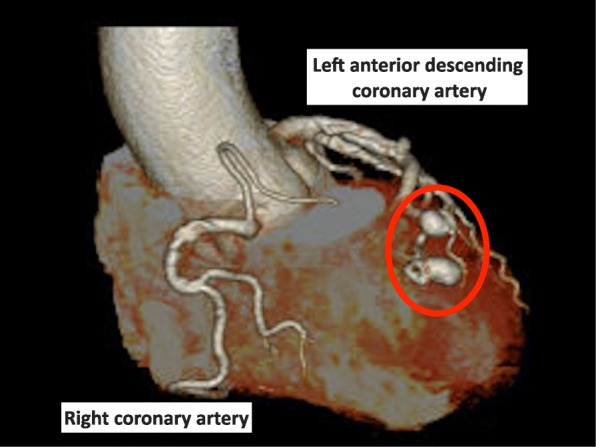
Preoperative coronary 3D-CT: Two coronary artery aneurysms are recognized in the periphery of the left anterior descending branch (red circle)
